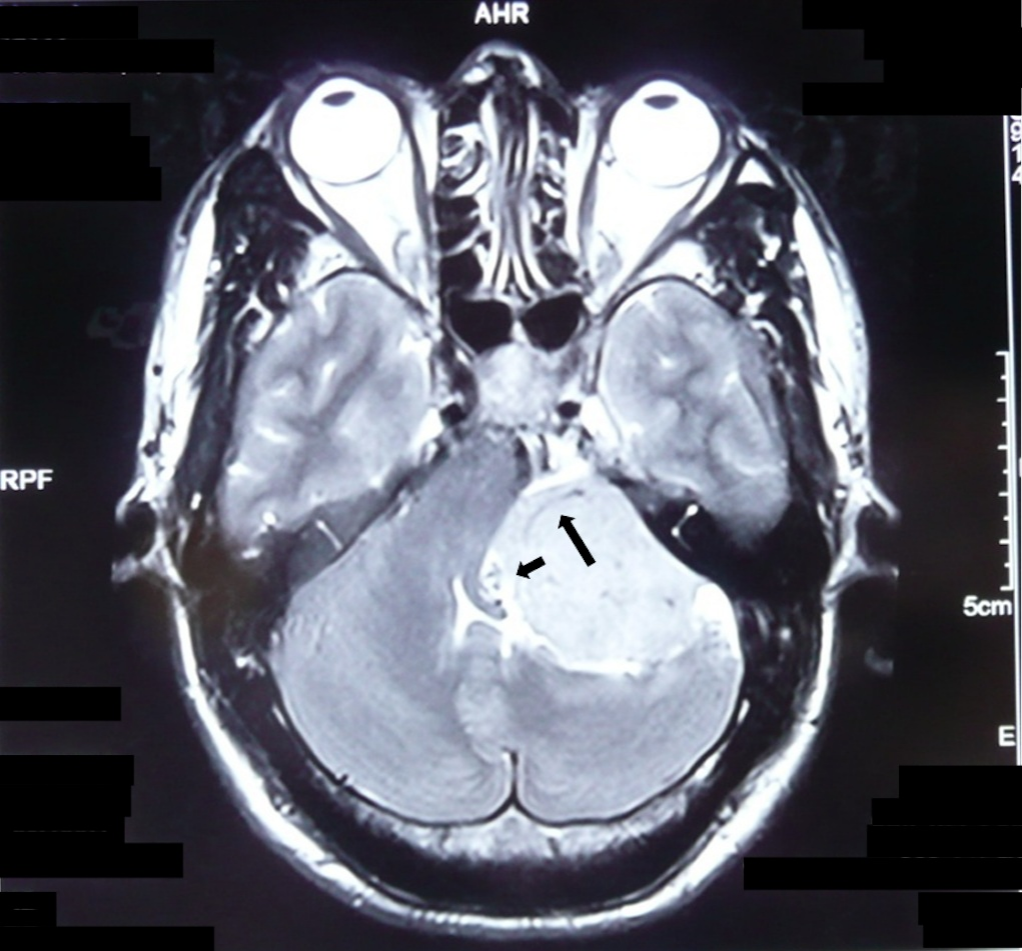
Feeding Vessels MRI with contrast demonstrating the blood supply to the meningioma in a 36-year-old male. This CPA meningioma had many adjacent vessels (short arrow) and perforating vessels (long arrow). CPA: cerebellopontine angle; MRI: magnetic resonance imaging
